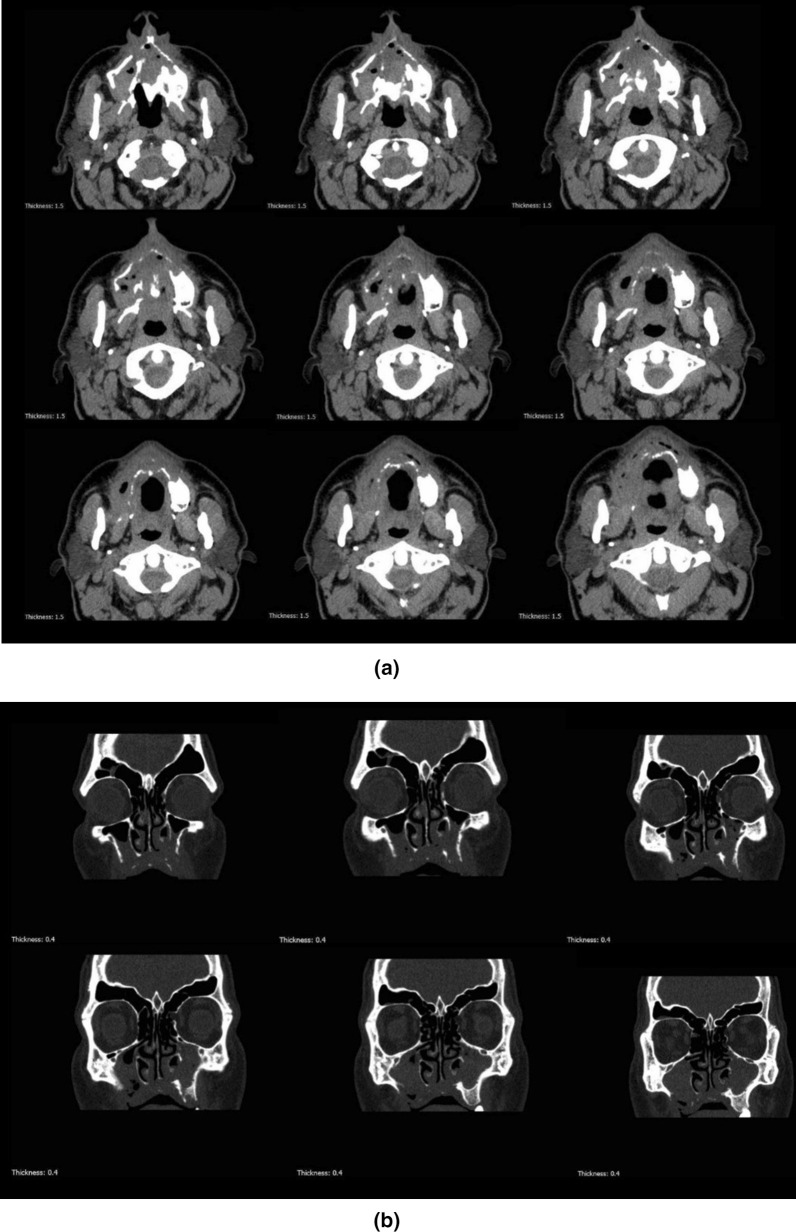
Axial (A) and coronal (B) view of computed tomography scan of the patient
